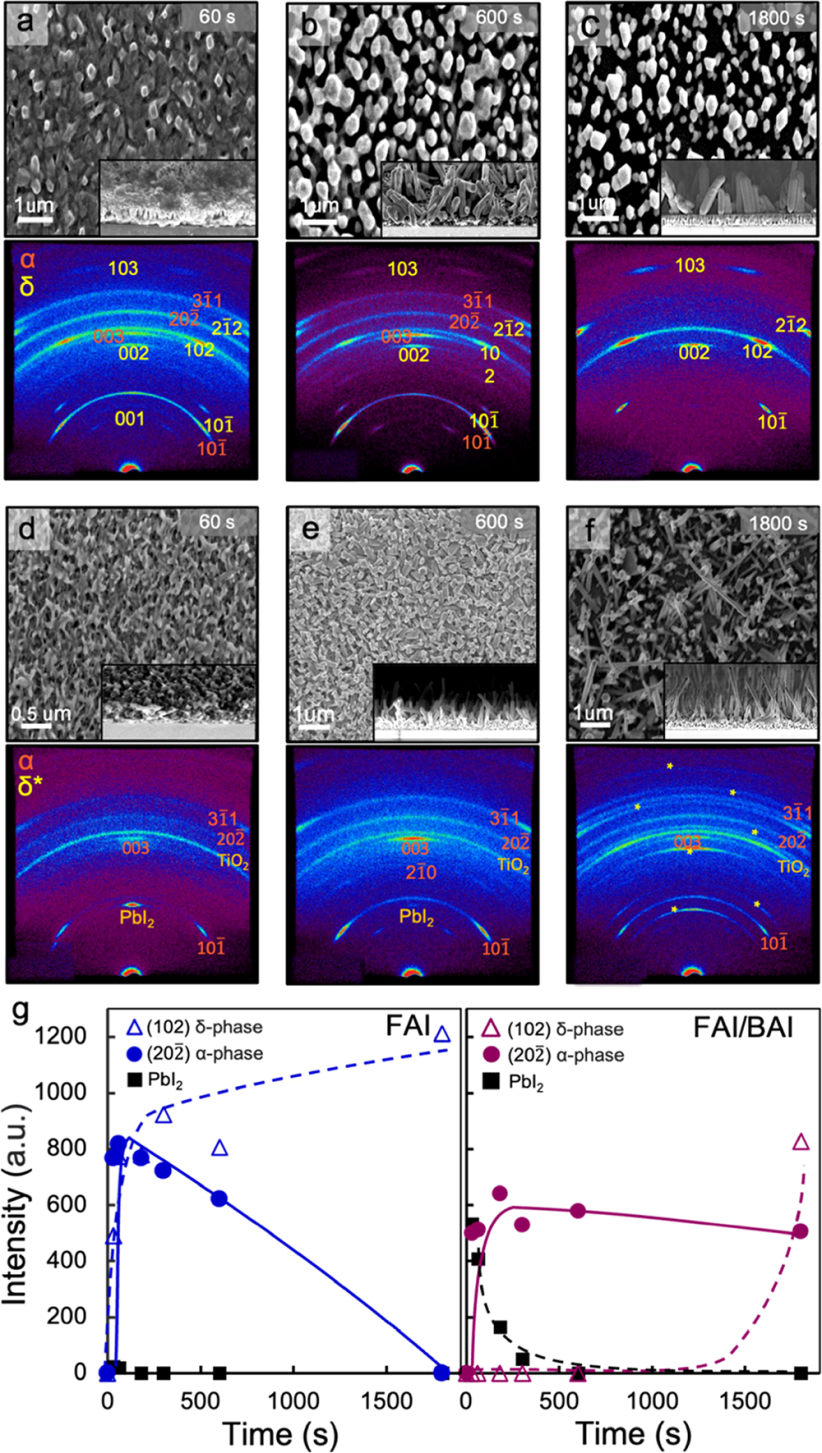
Top-view SEM images and corresponding 2D X-ray
